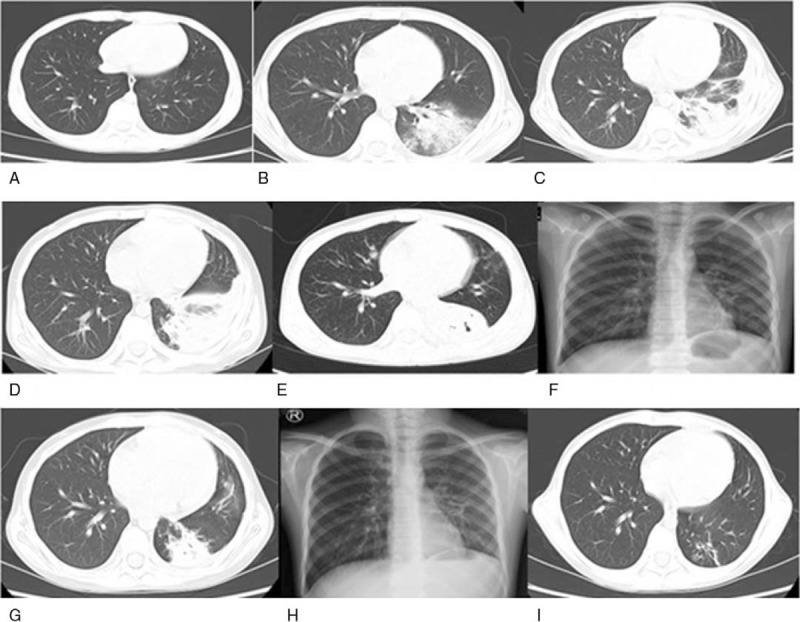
Chest CT findings in the whole process. A, On admission. B, Hemoptysis: pleural effusion with high-density areas in the left lower lobe. C, Voriconazole intravenously for 2 weeks. D, Voriconazole for 4 weeks. E, Aggravation of consolidation, atelectasis, necrosis, and cavitation in the lower left lobe after voriconazole for 12 weeks. F, Oral posaconazole for 1.5 months. G, Oral posaconazole for 3 months. H, Oral posaconazole for 4 months. I, The lesions were obviously absorbed after posaconazole withdrawal for 3 months. CT = computed tomography.
